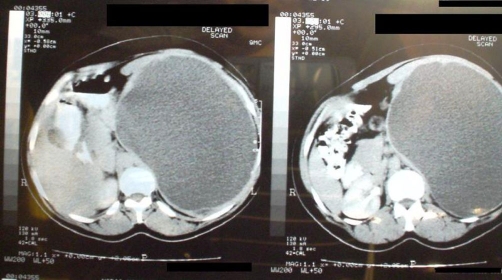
CT scan showing large splenic cyst reaching midline.
